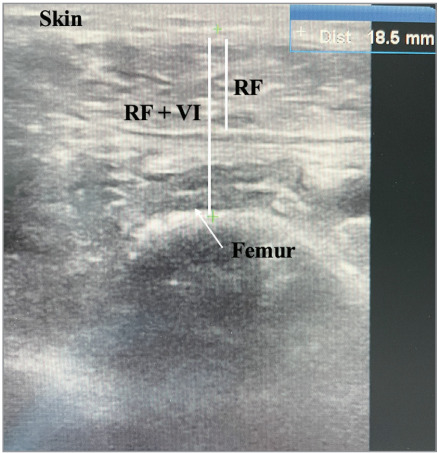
Ultrasound view of rectus femoris (RF) and vastus intermedius (VI) muscles in B mode.
